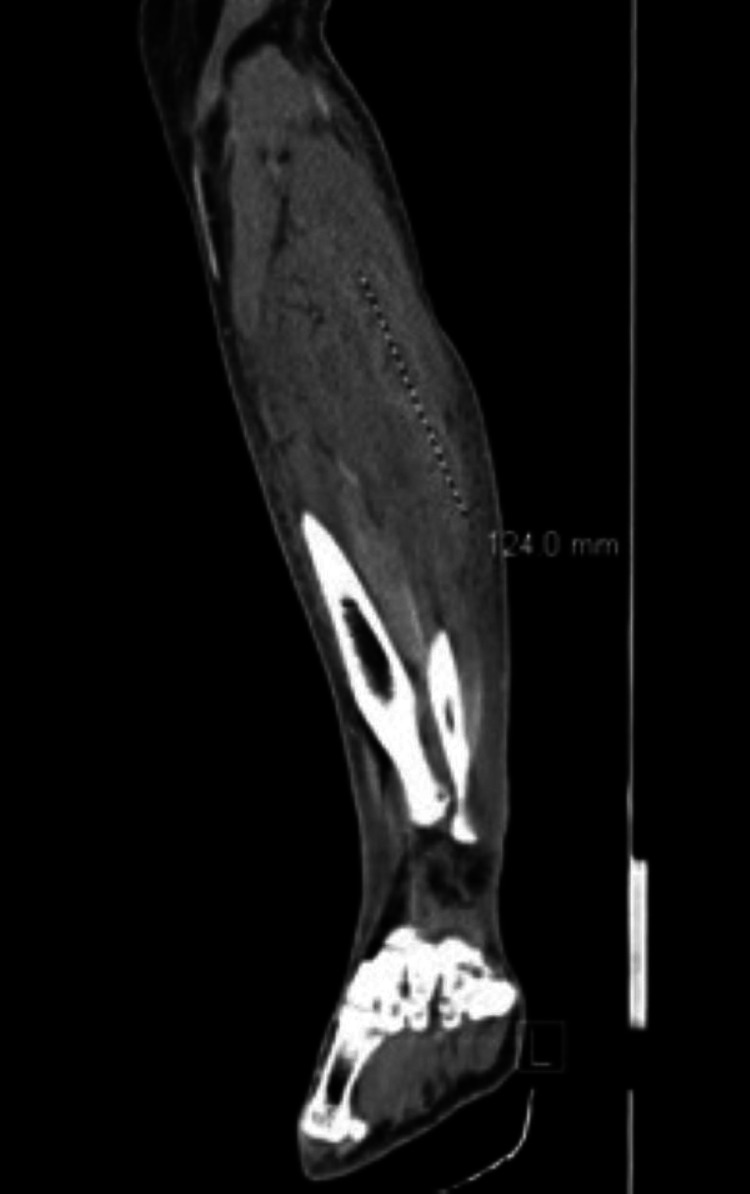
Left lower extremity CT with 12.4 cm fluid collection of the gastrocnemius visualized (depicted by dotted line), sagittal view.
